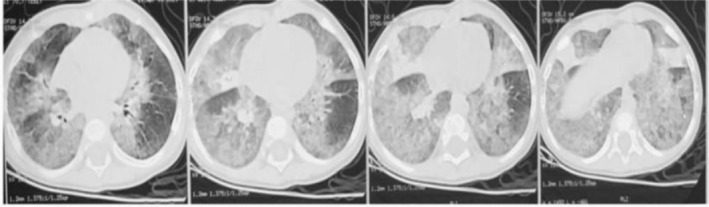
CT Chest: Bilateral diffuse ground glass opacities involving entire lung fields
